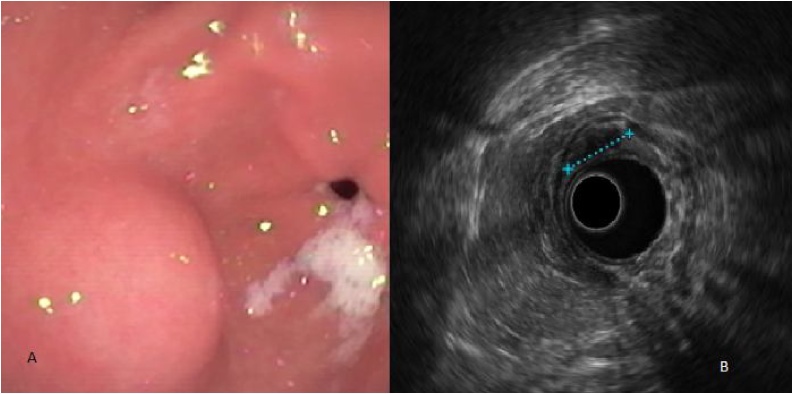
Sub epithelial gastric antrum lesion [A] in the second ultrasonographic layer of 25 mm [B].
